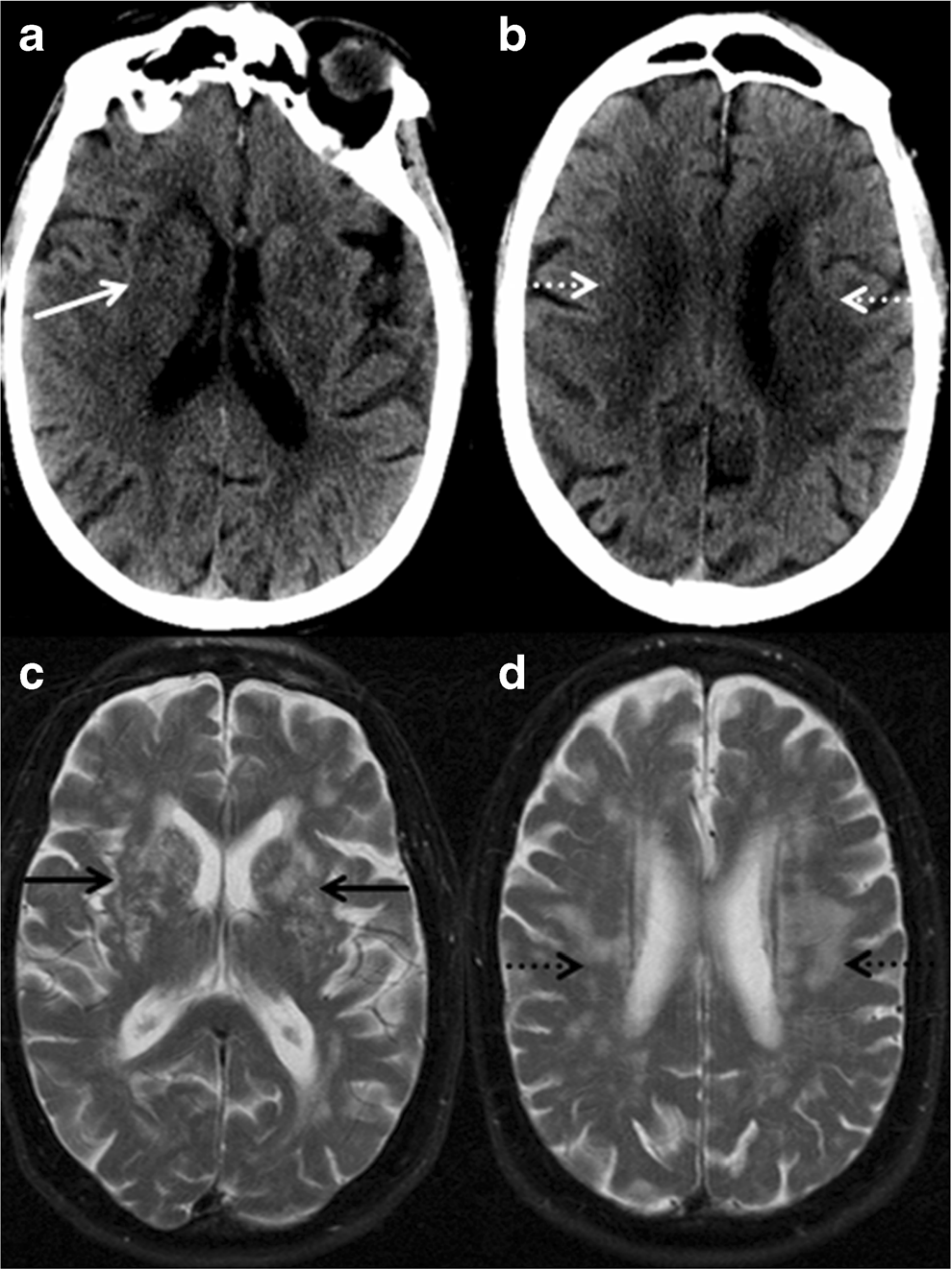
Vascular dementia. An 86-year-old man with multiple transient ischemic attacks (TIAs), ongoing changes in level of consciousness and memory. a & b noncontrast CT scan of the head shows multiple patchy confluent hypodensities in bilateral basal ganglia (*white arrow*), periventricular and subcortical white matter (*dashed white arrow*). c & d T2W MRI images of the brain revealing diffuse patchy and confluent hyperintensities in bilateral basal ganglia (*black arrows*) and white matter (*dashed black arrow*)
